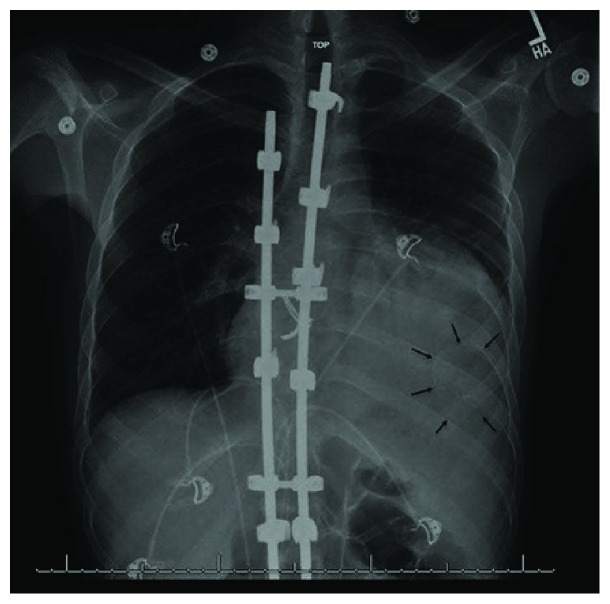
Chest radiography showing left lower lobe consolidation, cardiomegaly, and a calcified mass in the left lung base.
